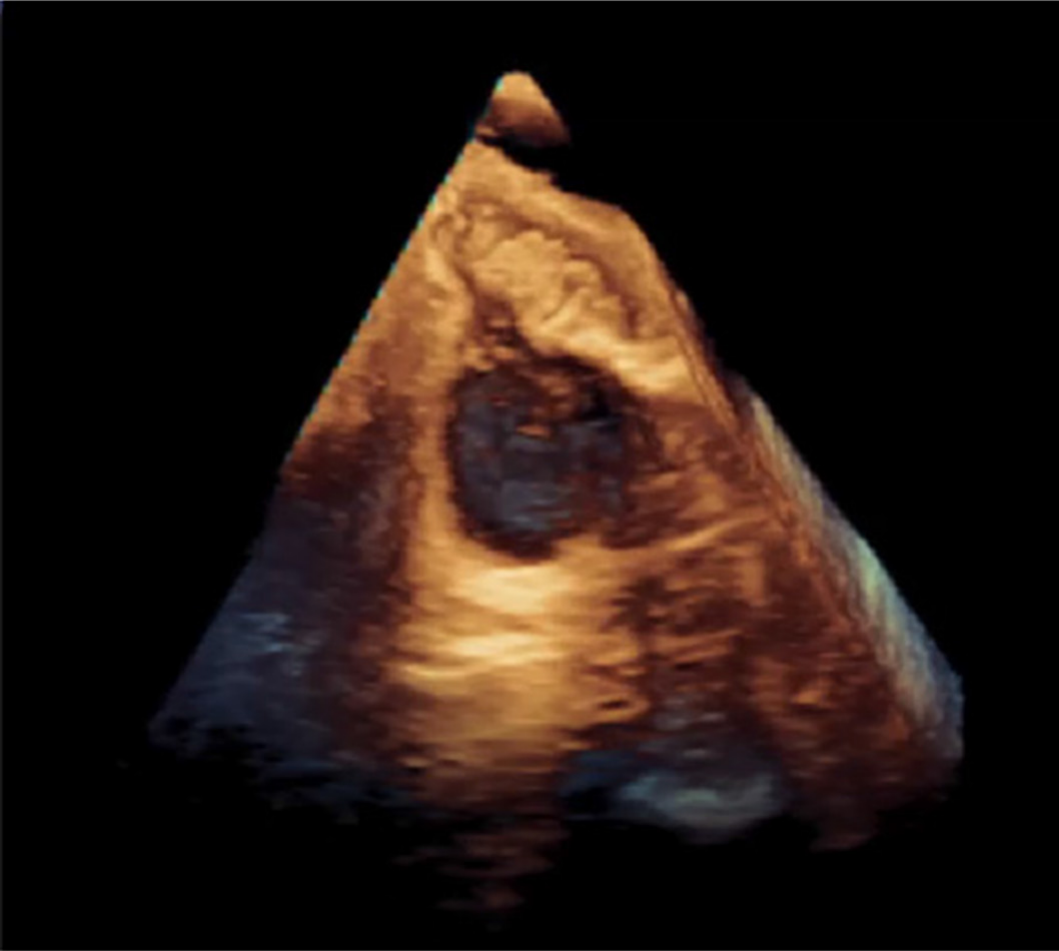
(Video S1): The 3D transesophageal echocardiography in the midesophageal 110° (live 3D image) shows the elongated irregular hypermobile spiral‐shaped mass in the right atrium.
